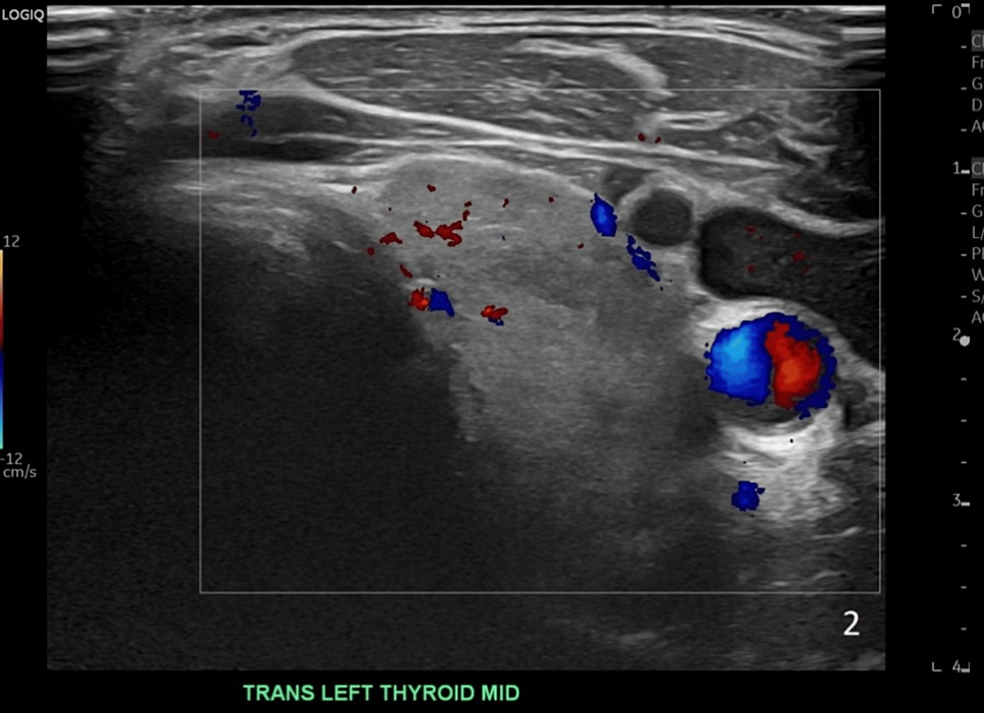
Image of the left lobe thyroid ultrasound
